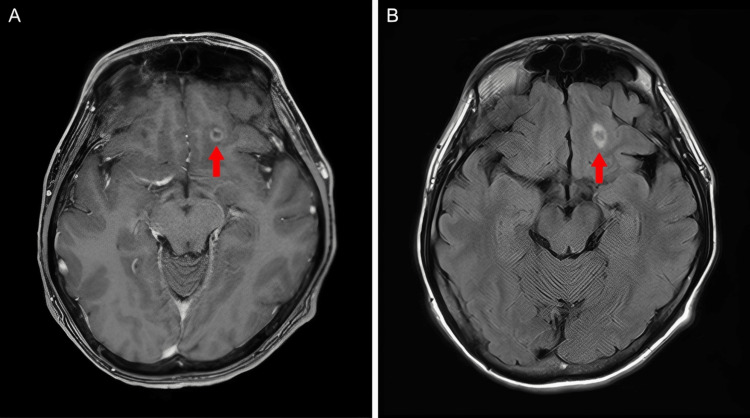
MRI findings Contrast-enhanced T1-weighted imaging demonstrated a ring-enhancing lesion in the left frontal lobe (A, arrow). FLAIR imaging showed perilesional edema associated with the lesion (B, arrow). FLAIR: fluid-attenuated inversion recovery
